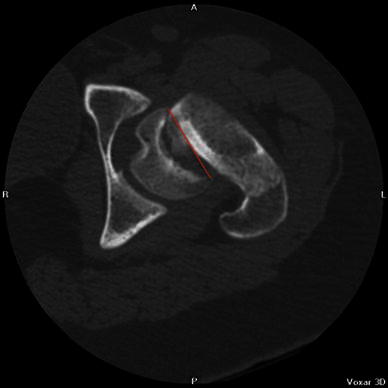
A line is drawn across the corners of the epiphysis on an axial computed tomography (CT) slice of the hip
